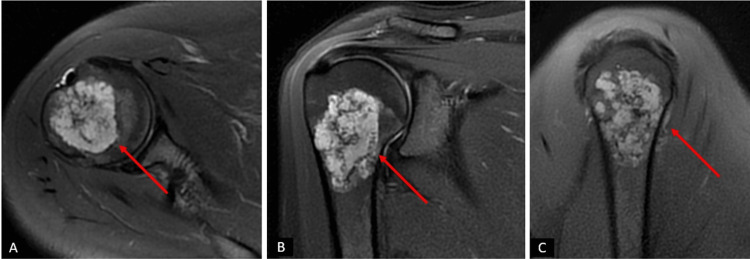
Preoperative magnetic resonance imaging (MRI) scans of the patient of right shoulder. (A) Axial section MRI images of the patient. (B) Sagittal section MRI images of the patient. (C) Coronal section MRI images of the patient. The regions in the proximal humerus with a preliminary diagnosis of enchondroma/low-grade chondrosarcoma are indicated with red arrows.
